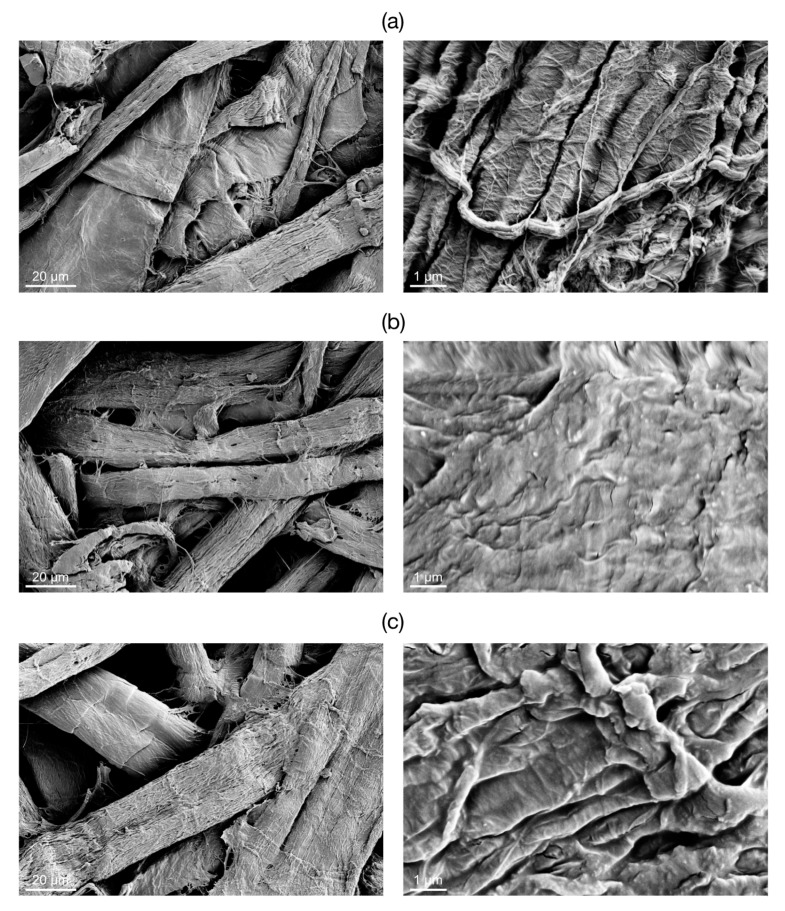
Low (left) and high (right) magnification SEM images of (a) the raw paper substrate and *Paper-NBB80h* samples, before (b) and after (c) rinsing in ultrasound bath. Images on the left were taken at the same level of magnification of those in Figure 1; images on the right were taken at a constant higher level of magnification.
